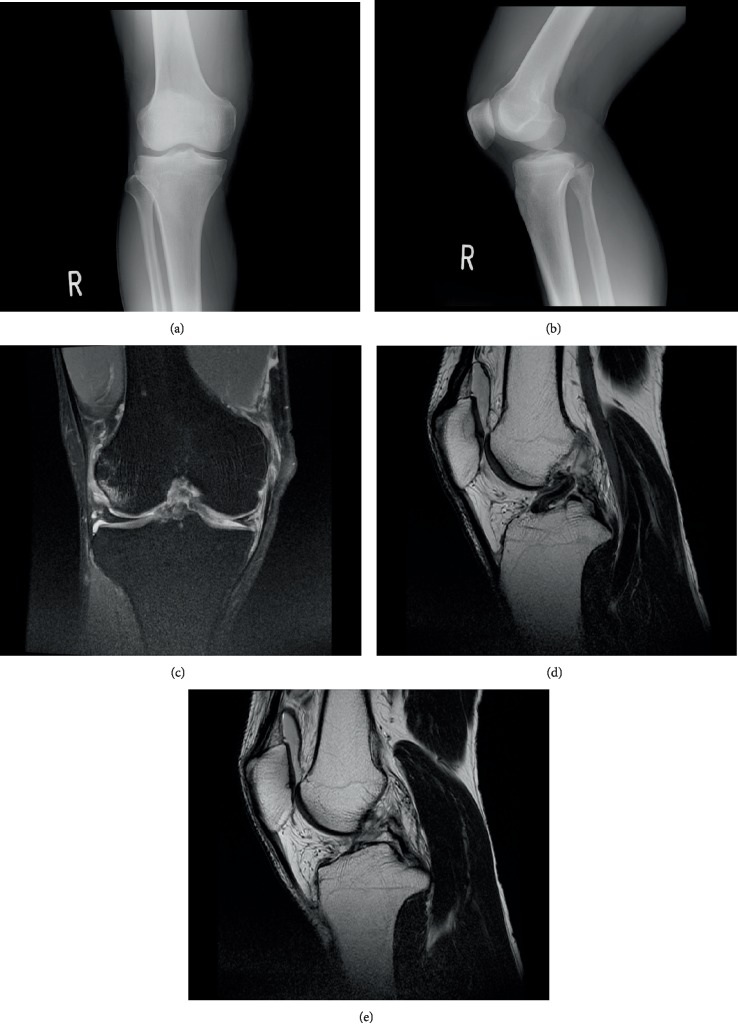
Preoperative images. (a, b) Preoperative anterior-posterior and lateral plain radiographs of the injured knee. (c) Preoperative coronal fat suppressed proton density-weighted magnetic resonance imaging (MRI) showing the proximal tear in the medial collateral ligament. (d, e) Preoperative sagittal proton density-weighted MRI showing the proximal tear in the anterior cruciate ligament (ACL) and posterior cruciate ligament (PCL).
